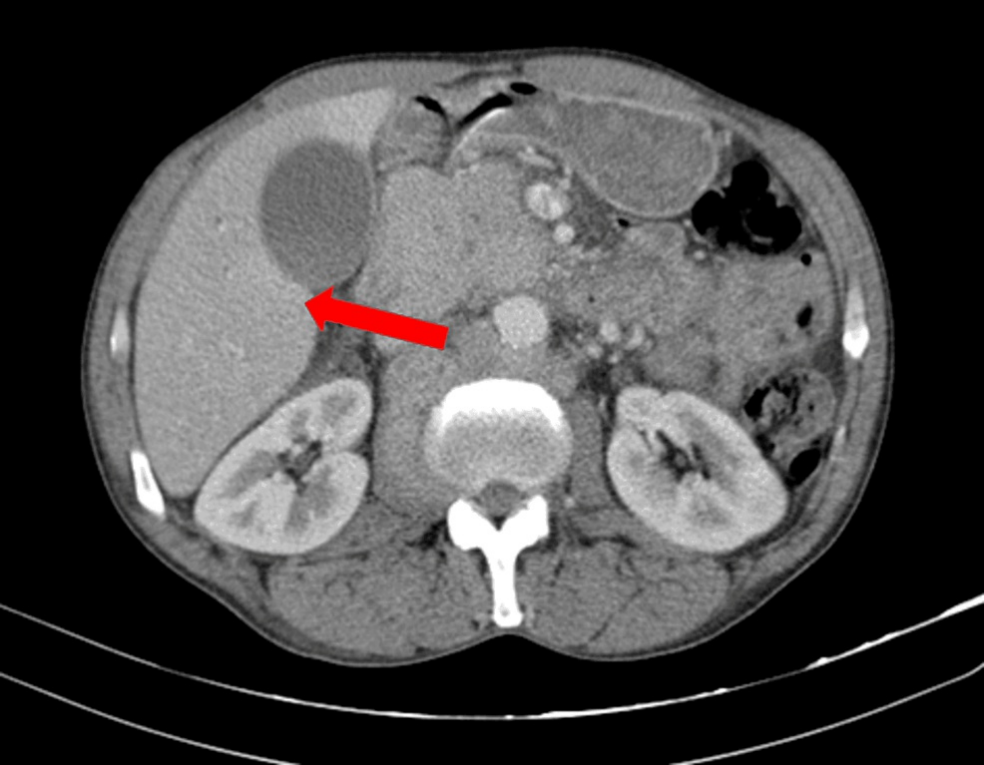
Abdominal CT scan. Liver and pancreas globose, with preserved structure. The pancreas exerts a compressive effect on the hepatic hilum and causes dilation of the bile ducts.
